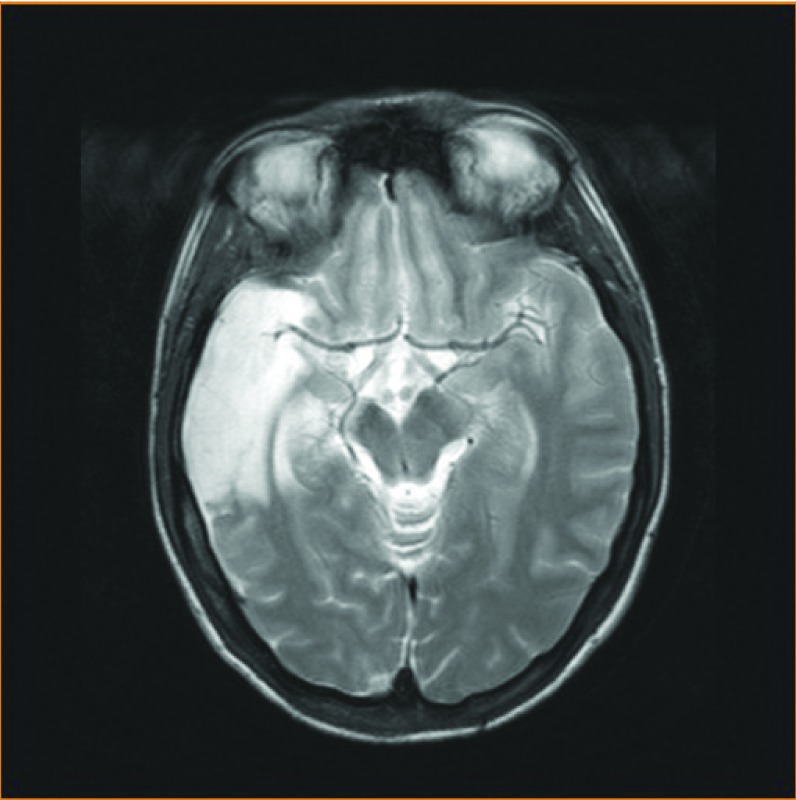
MRI sequence of a severe lesion in the area subtended by the middle cerebral artery. The injury was sustained before PFO closure, most probably due to paradoxical embolism after documented deep venous thrombosis in a 25-year-old woman.
